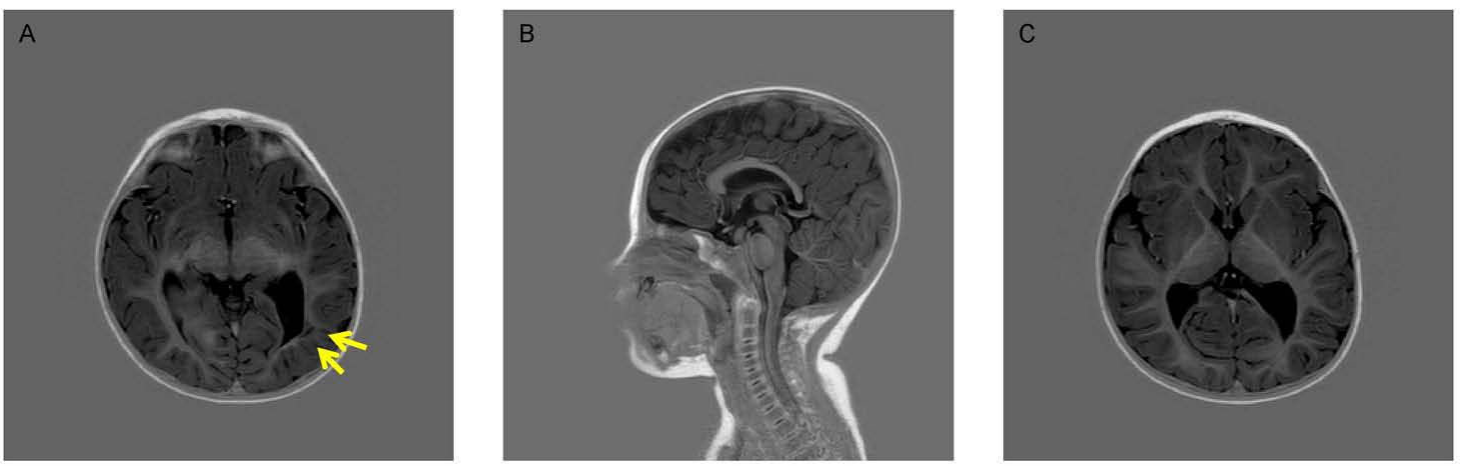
Brain MRI showing (A) possible brain atrophy and possible hemorrhage, (B) slight thinning of the corpus collosum posteriorly and (C) decrease in white matter volume seen posteriorly in the cerebral hemispheres bilaterally.
